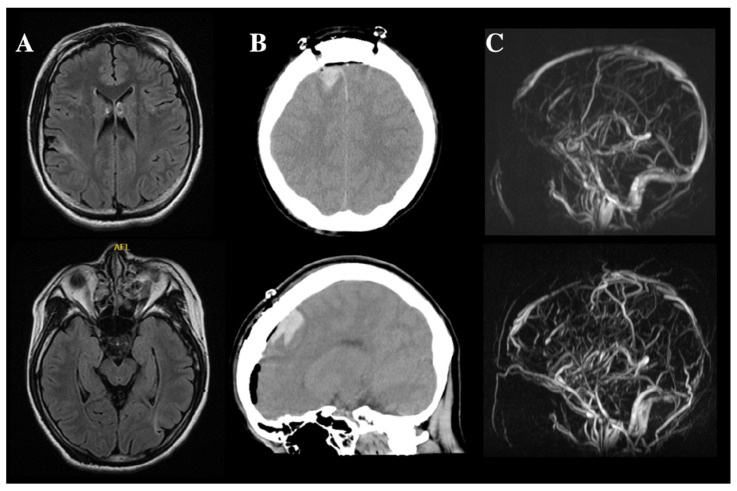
MRI and CT of the patient with anti-NMDAR encephalitis after CC. (A) MRI before CC revealed cortical scars at the right postcentral gyrus and left occipital lobe. (B) CT the day after surgery showed cerebral hemorrhage around the right supplemental motor area. At this time, mild edema was also apparent at the left frontal lobe. (C) Superior and inferior images represent pre- and postoperative MRA, respectively. The superior sagittal sinus and straight sinus were not evident on postoperative MRA.
